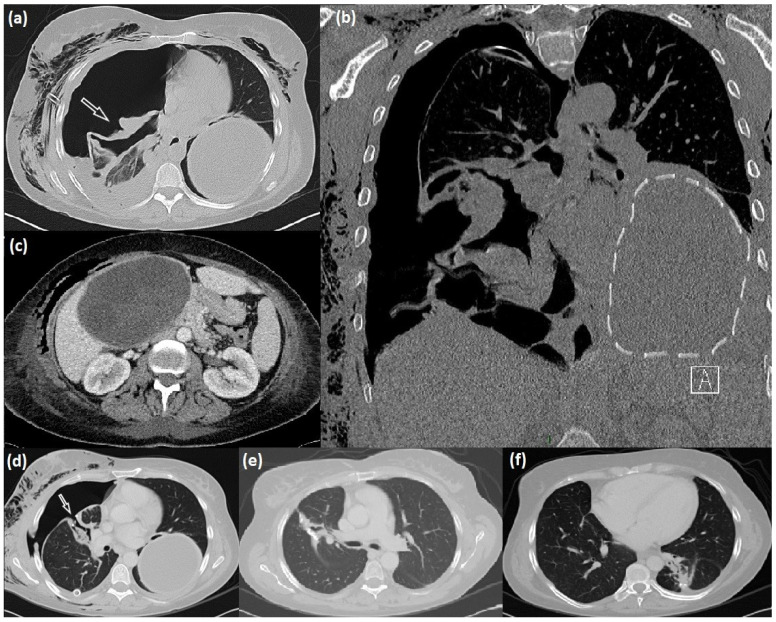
Radiological manifestation of lung-liver hydatidosis. (a–c) CT scan appearance of the right lung ruptured cyst (arrow), intact giant left lung cyst (dotted line), and liver cyst; (d) right bronchopleural fistula (arrow) after cystectomy, and after its surgical repair (e); postoperative results after left cyst surgical excision (f).
